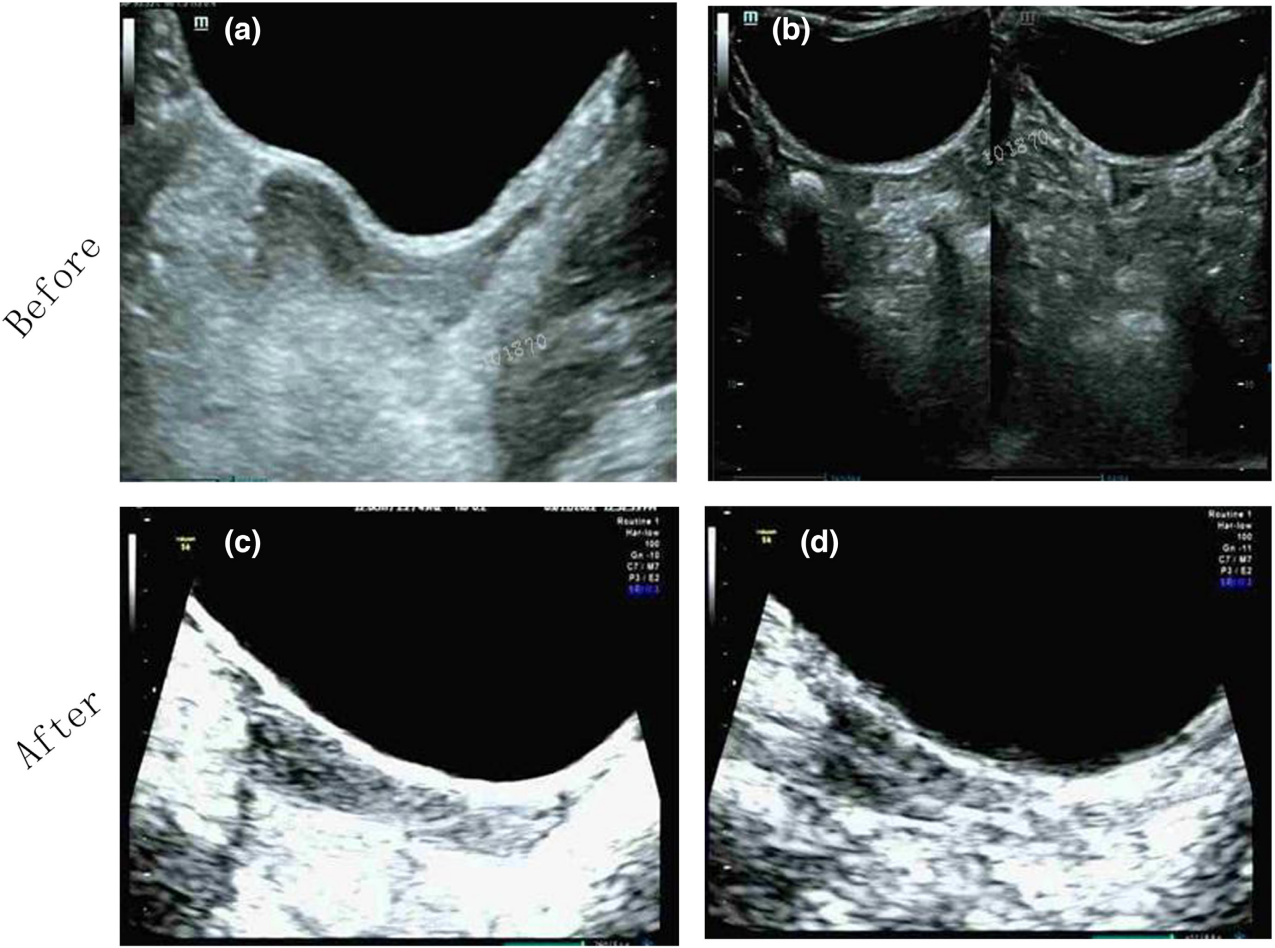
Pelvic ultrasound before and after laparoscopic bilateral gonadectomy. (a) Pelvic ultrasound image prior to laparoscopic bilateral gonadectomy depicting the uterus. (b) Pelvic ultrasound image prior to laparoscopic bilateral gonadectomy revealing both gonads. (c) Pelvic ultrasound image post laparoscopic bilateral gonadectomy and after 3 months of puberty induction, displaying the uterus. (d) The endometrium appears linear following 3 months of puberty induction.
